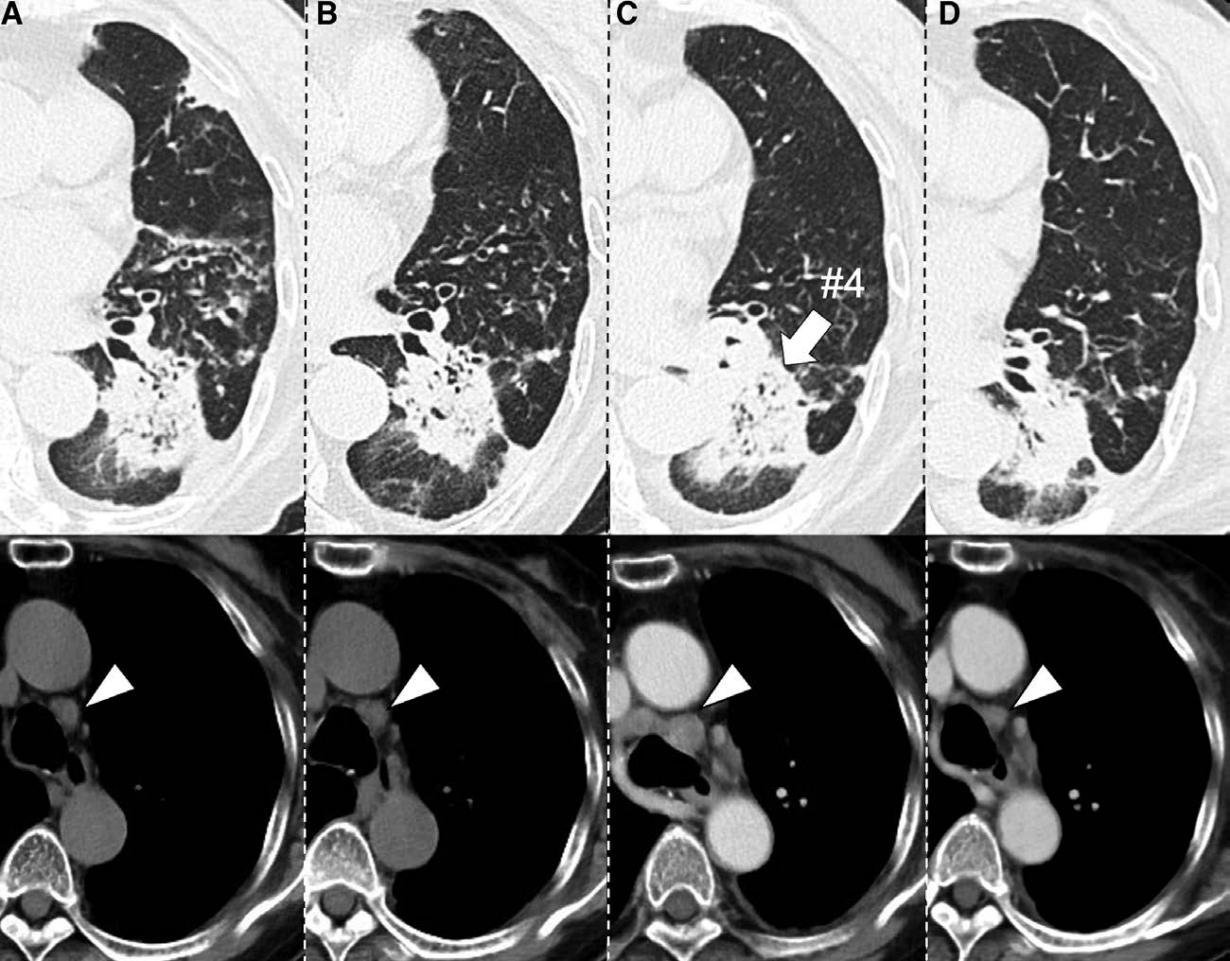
(A and B) At the current admission, chest computed tomography (CT) images showed an exacerbation of left S8 consolidation (A), and increasing PSL dose (30 mg/d) resulted in a partial improvement of consolidation (B). (C) CT images showed deterioration of consolidation at the time of adenocarcinoma diagnosis. TBLB performed targeting #4 (arrow). (D) 10 months of treatment with alectinib resulted in a partial response. Arrowheads indicate lymphadenopathy during the course. PSL = prednisolone, TBLB = transbronchial lung biopsy.
